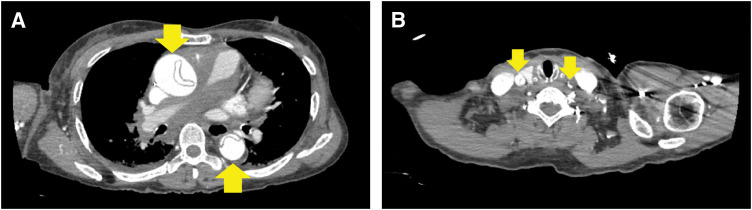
CT with venous enhancement. (A) Dissection from the ascending aorta (yellow arrows). (B) Dissection of both common carotid arteries; the left was almost completely blocked (yellow arrows).
